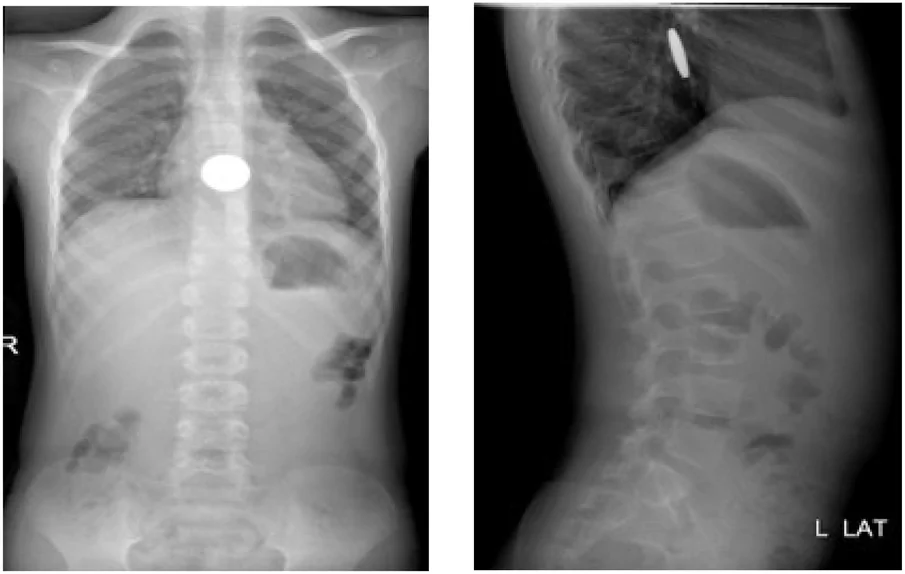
Initial posterior-anterior (A) and lateral (B) radiographs showing a radio-opaque round object lodged in the lower third of esophagus.
